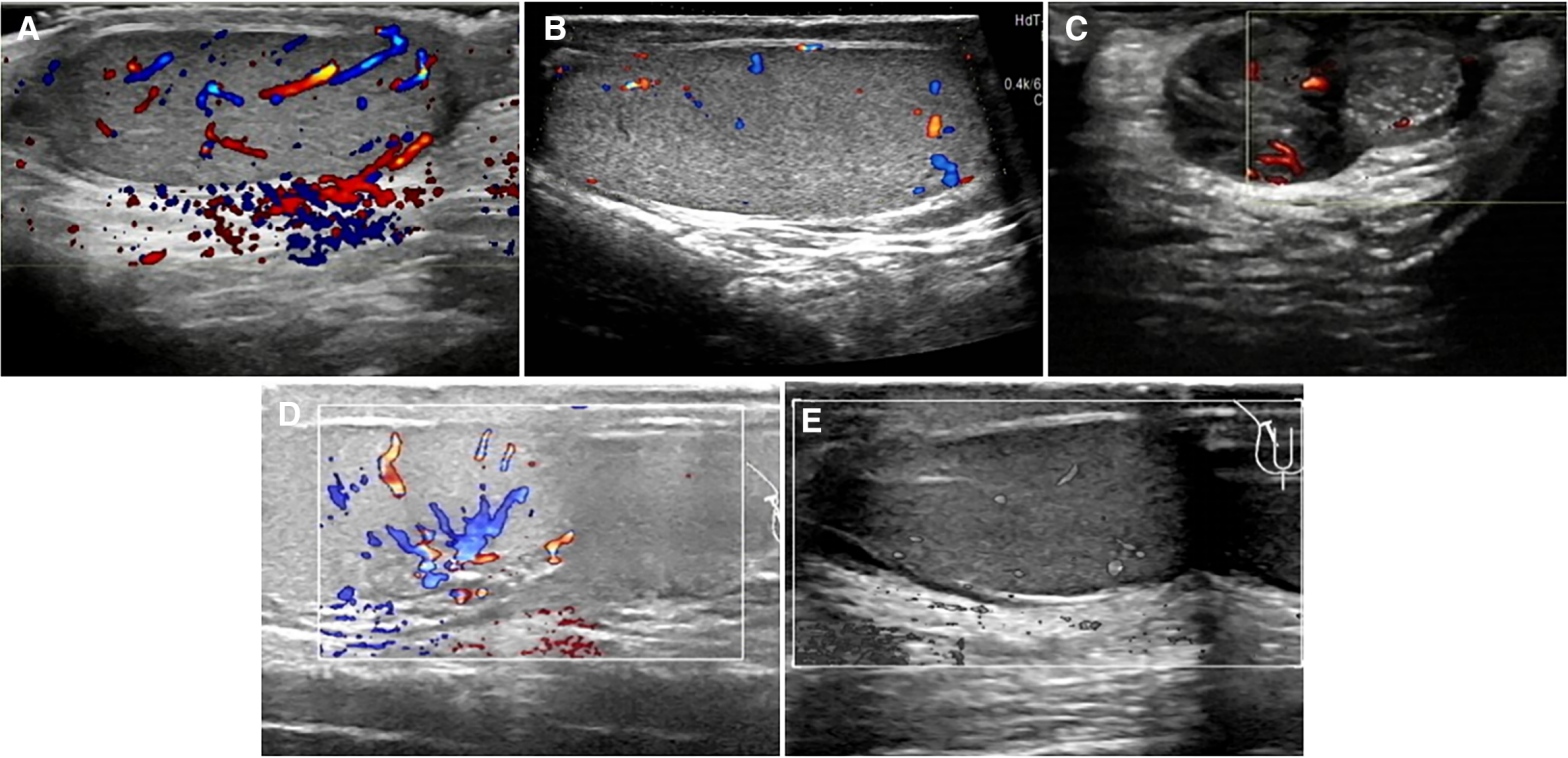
Postoperative ultrasonographic findings of torsional testes in five cases. (A) Uniform internal echo in the testis; CDFI: normal blood flow signals in the testis, stellate blood flow signals in the tunica albuginea in case 2. (B) Uniform internal echo in the testis; CDFI: normal blood flow signals in the testis, stellate blood flow signals in the tunica albuginea in case 3. (C) Testis presented uneven internal echo with slightly punctate stronger echo; CDFI: several blood flow signals around the diseased testis but no obvious signals within it in case 4. (D) Testicular parenchyma echo was uniform; normal echo was observed around the testis; CDFI: normal blood flow signals were found in the testis in case 7. (E) Internal echo of the testis was uniform; no cystic or solid foci were observed; CDFI: normal blood flow signals in the testis in case 8.
